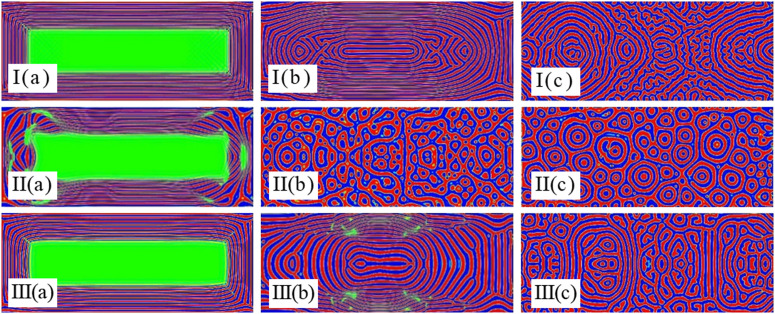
Coupling effect of the chemical reactions and ultrasonic fields on steady patterns with φ=0.5, $K=10^{-4}$ at times (a)t=1732×8, (b)t=1732×20 and (c)t=1732×100 for (I) f=0.01MHz\nolimits, (II) f=1MHz\nolimits and (III) f=10MHz\nolimits.
